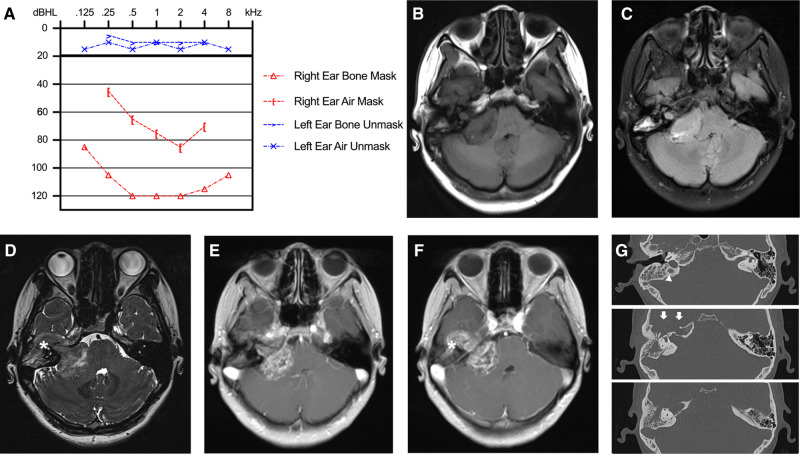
Preoperative hearing and imaging scans. (A) Preoperative pure tone threshold audiometry demonstrated profound mixed hearing impairment of the right ear. (B–F) MRI scan revealed a mass with a maximal diameter of 5.0 cm at the right cerebellopontine angle with petrous bone invasion. The lesion exhibited low- to iso-intense on T1-weighted images (B), iso- to high-intense on T2-FLAIR images (C), mixed intense on constructive interference in steady state (CISS) images (D), and heterogeneously enhanced after Gd-DTPA administration (E,F). (G) In the CT scan, we observed an enlargement of the right IAC with significantly bony destruction of the temporal apex (white arrows) and a slightly high ride of the jugular bulb (white triangle).
